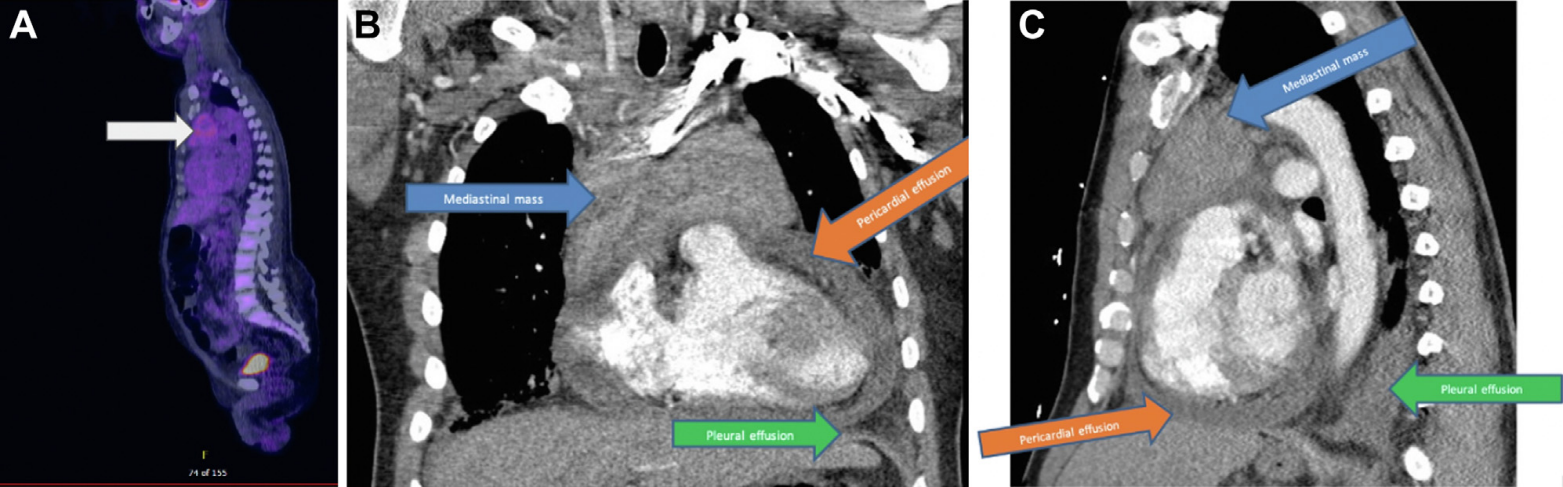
(A) Sagittal chest computed tomography (CT). (B) Coronal chest CT revealing mediastinal mass (blue arrow), pericardial effusion (orange arrow), and pleural effusion (green arrow). (C) Sagittal chest CT.
